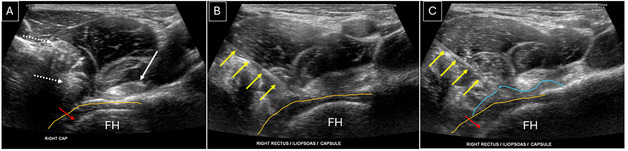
Hip pericapsular injection. Transverse ultrasound image of the proximal hip joint, just distal to the ilium (A). The iliopsoas (solid white arrow) and rectus femoris tendon (dashed white arrows) are also visible in short axis. During injection, the needle (yellow arrows) is advanced up to the hypoechoic joint capsule (orange outline) at the level of the femoral head (FH) at the level of the femoroplasty (red arrow) (B). The therapeutic anesthetic and steroid mixture (blue outline) is then injected, taking care to avoid intra‐articular injection (C).
